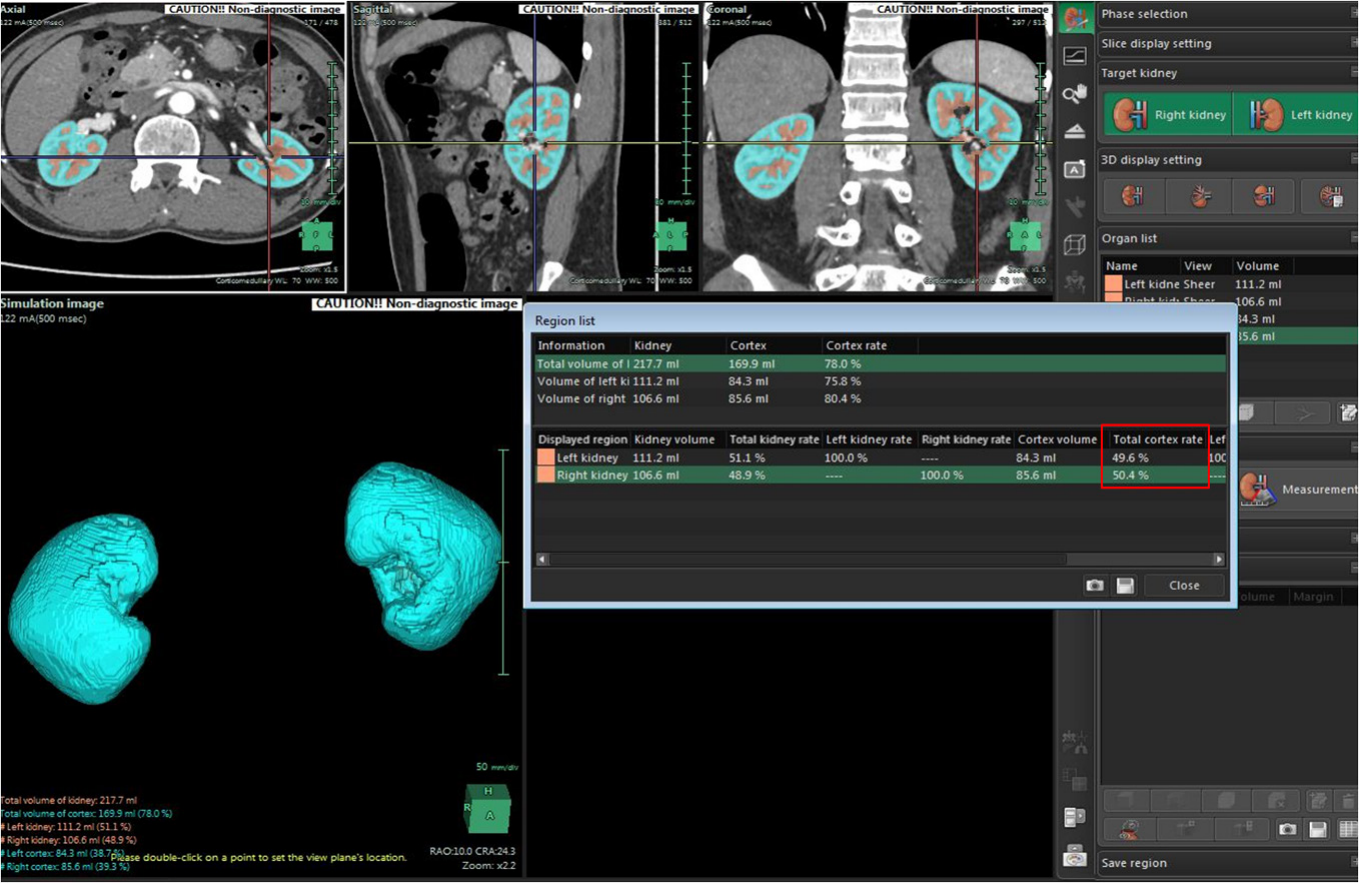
The software estimated the RCV on corticomedullary CT images. RCV, renal cortical volume.
